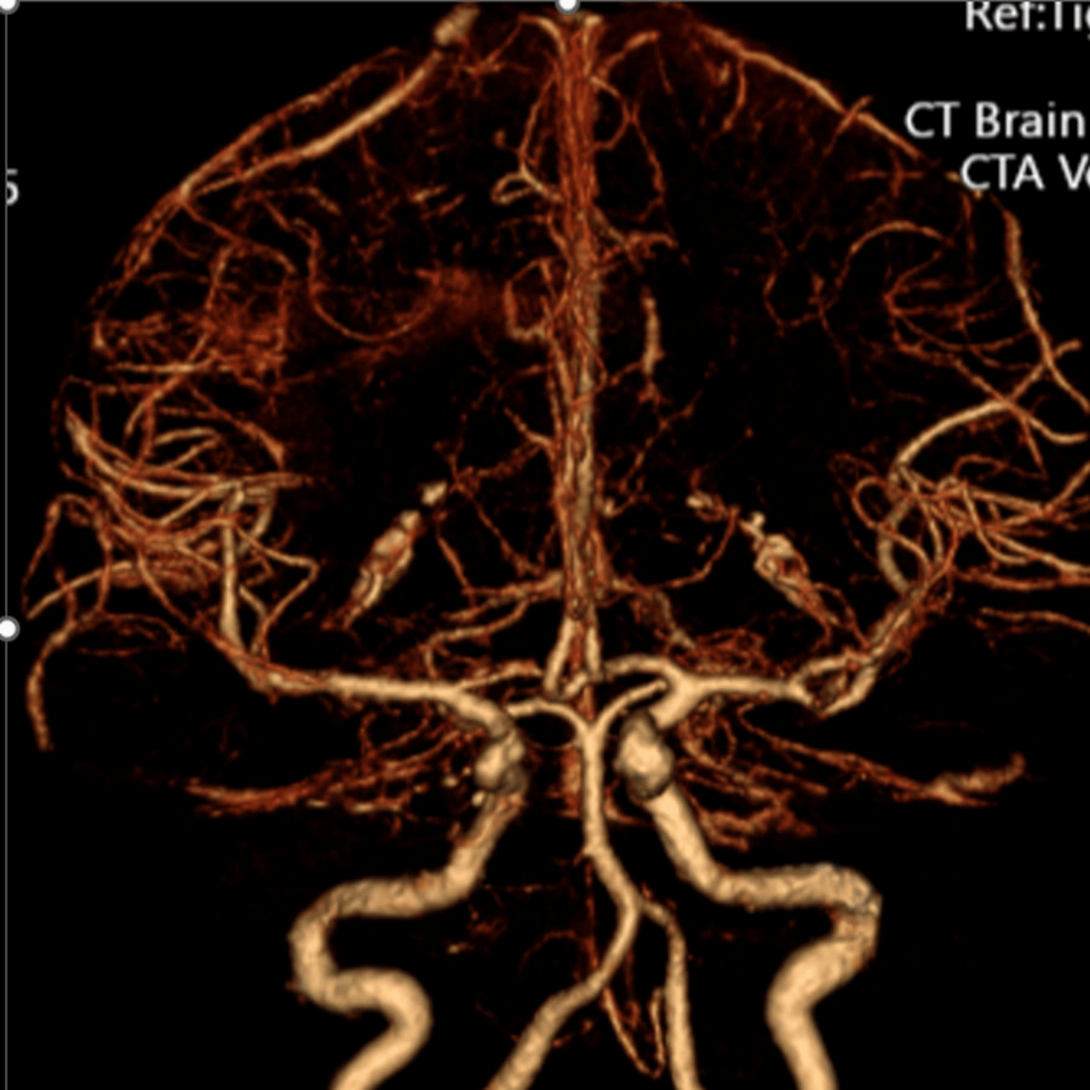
CTA head with contrast CTA: computed tomography angiography CTA of the head with contrast demonstrating normal intracranial vasculature. There is no evidence of large vessel occlusion, critical stenosis, aneurysm, or traumatic pseudoaneurysm
